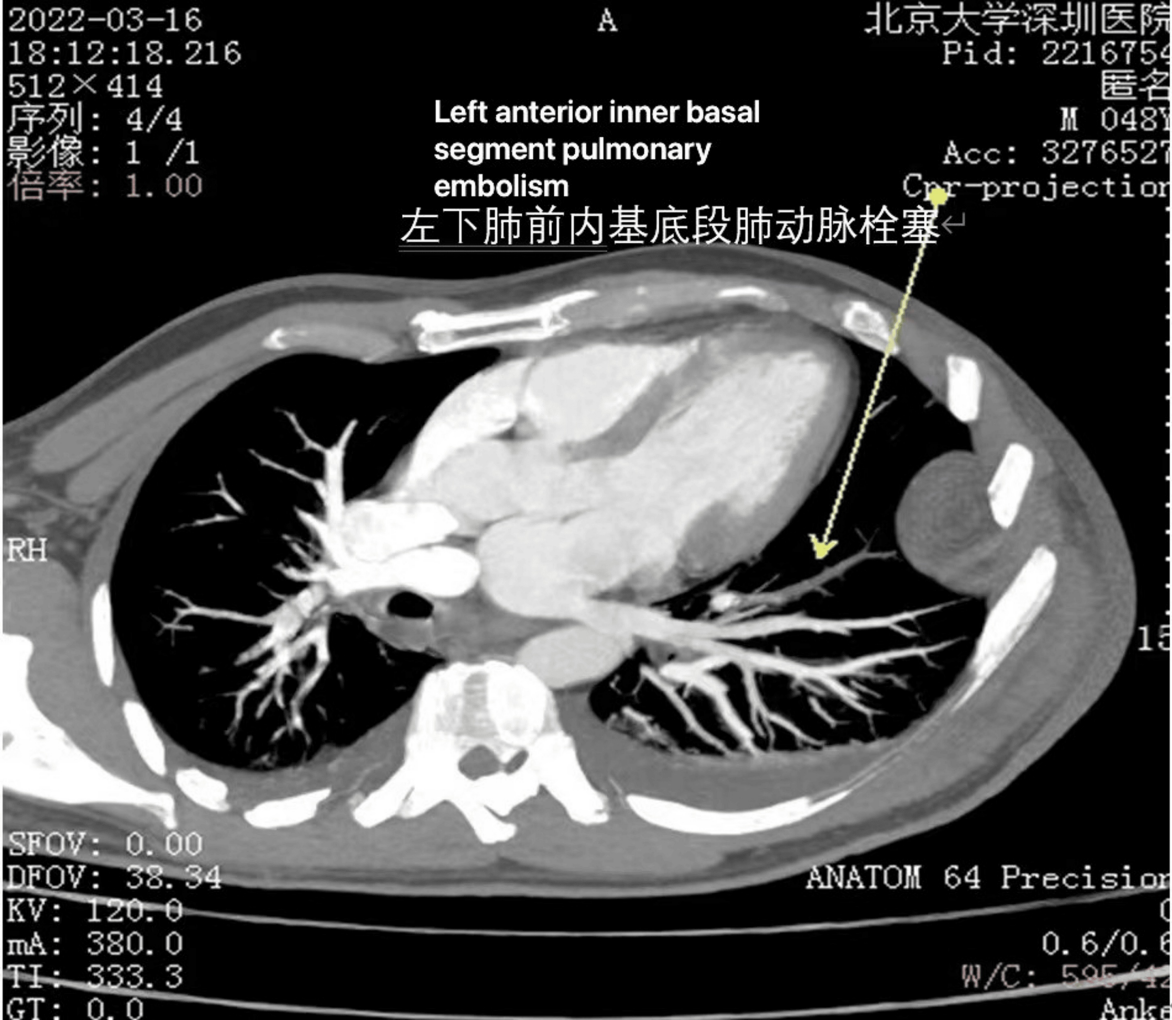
Computed tomography angiography (CTA) of Case 2 showing pulmonary embolism (PE) (arrow)
